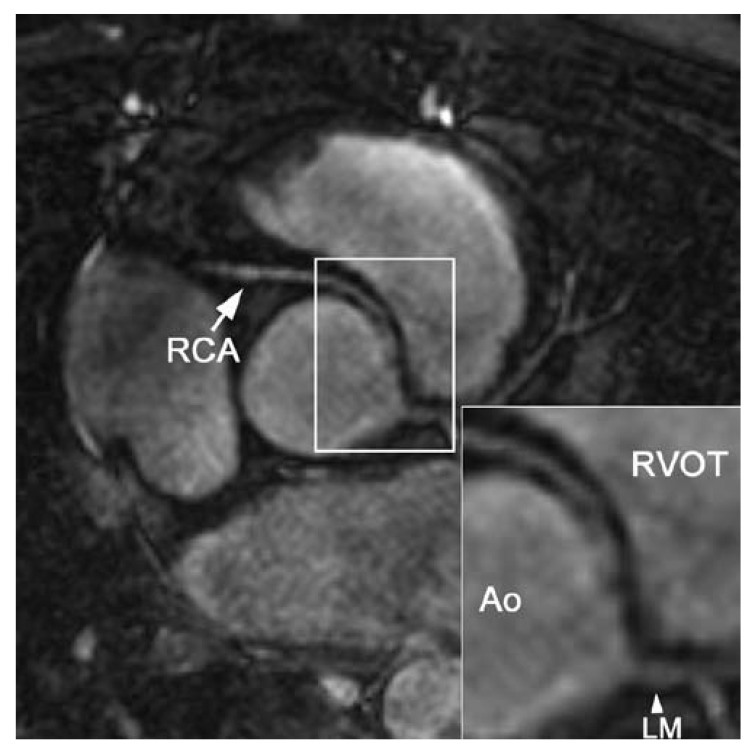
Three-dimensional balanced steady-state free precession (bSSFP) coronary MRI
